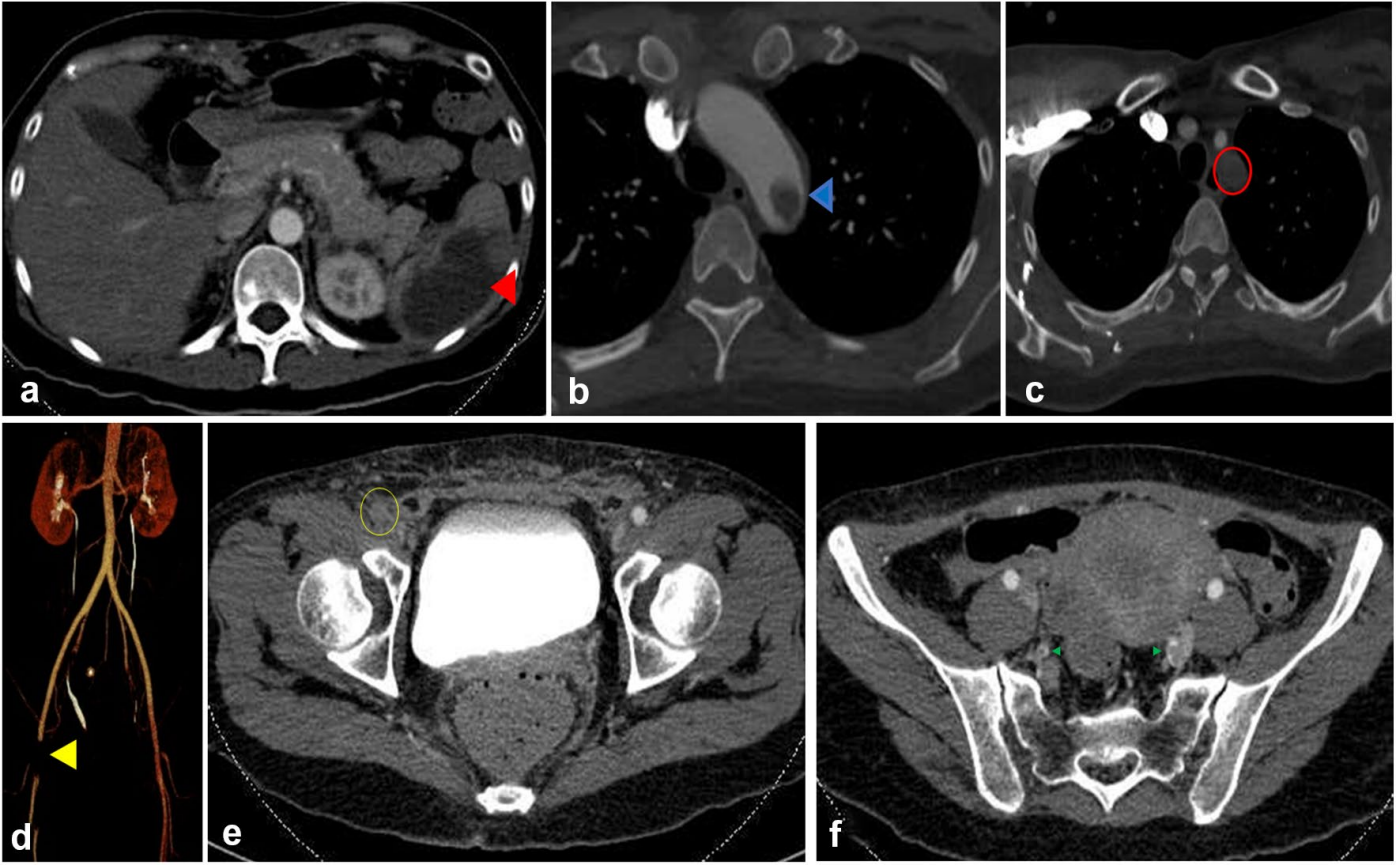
Computed tomography angiography images. a Spleen infarction (red triangle). b Thrombosis of the thoracic aorta (blue triangle). c Subclavian artery thrombosis (red circle). d Left common femoral artery embolism (yellow triangle). e Left common femoral artery embolism (yellow circle). f Bilateral internal iliac artery embolism (green triangle)
